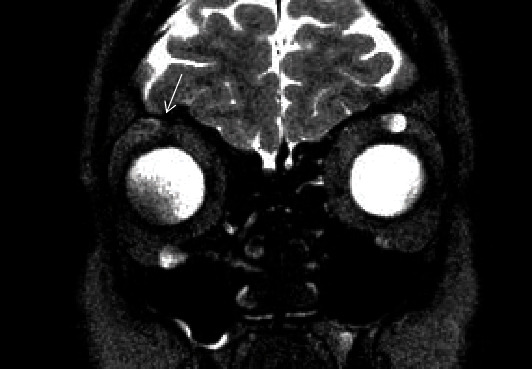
Postoperative coronal RMI T2-weighted imaging showed no recurrence in superior orbit right side (arrow). There was discrete increase in size of those located in the inferior right orbit and in the superior and inferior left orbit.
